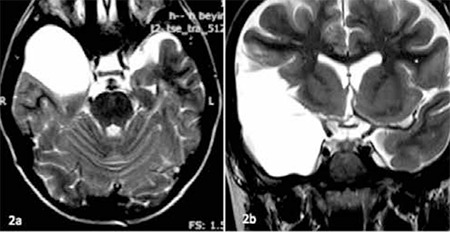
a,b) Axial/ Coronal MRI of patient 2 showing an extensive arachnoid cyst in the right middle cranial fossa, there was any compressive imaging findings (T2 weighted image)
